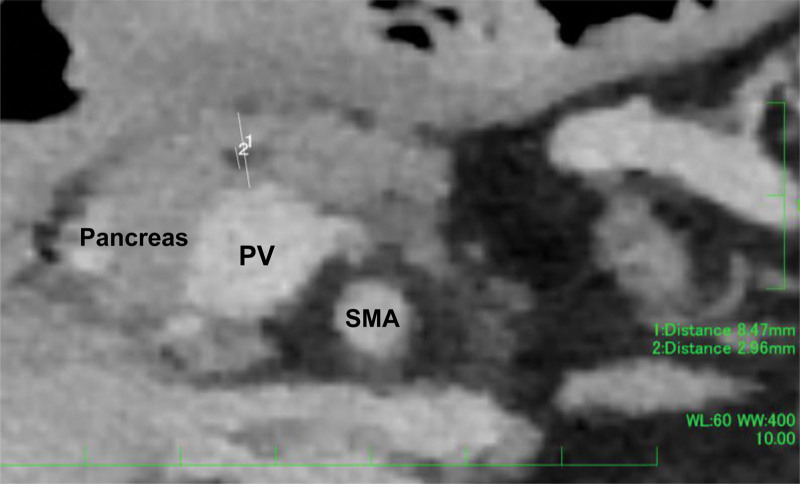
The dissection line during DP was confirmed based on the surgical record, and the thickness of the pancreas and the main pancreatic duct (MPD) diameter at the dissection line were measured using preoperative MDCT. DP = distal pancreatectomy, MDCT = multidetector computed tomography, MPD = main pancreatic duct.
